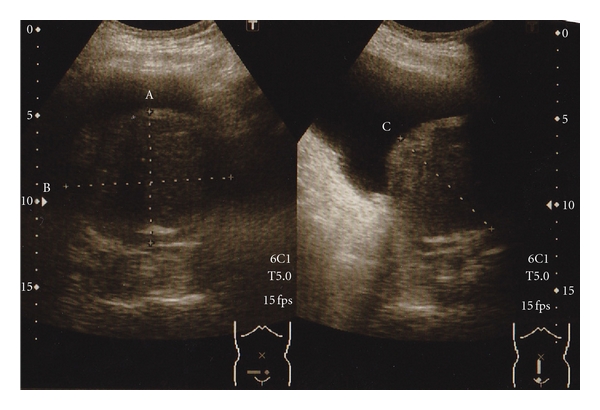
Transabdominal ultrasonography of large BPH in Case 1.
